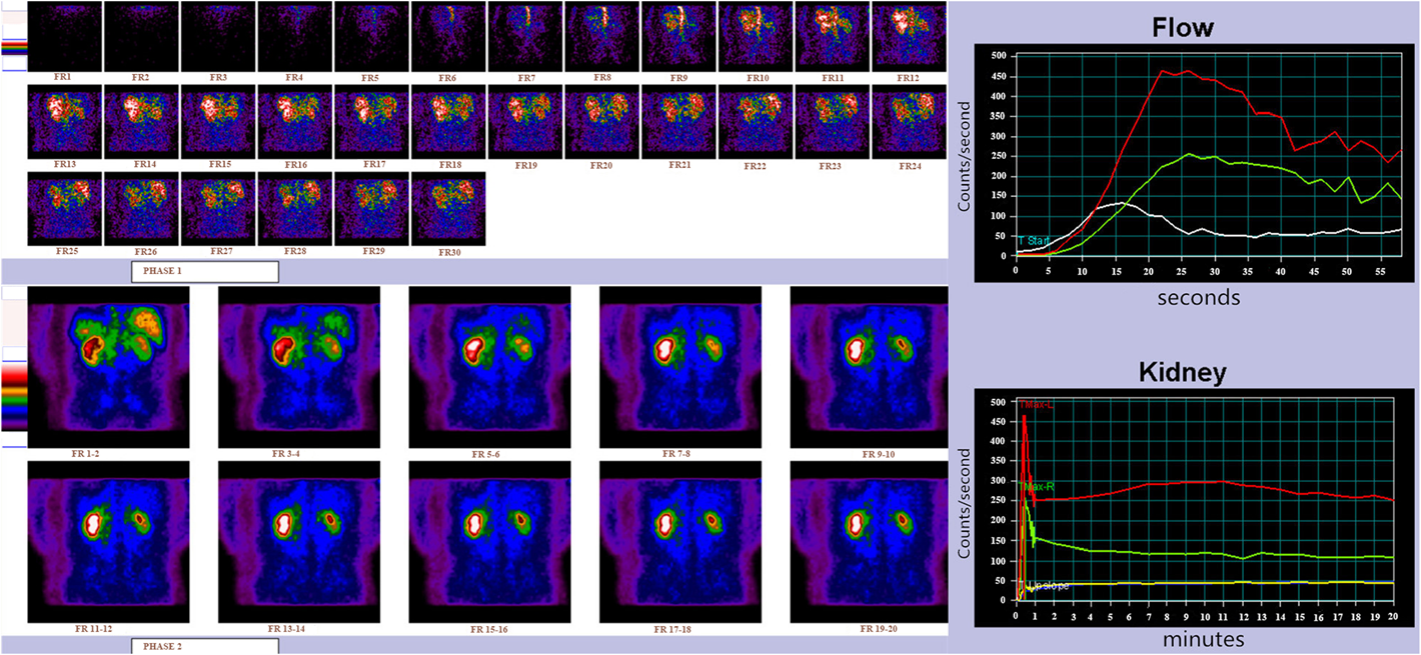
Dynamic renal scintigraphy for a critical patient by PICC. A 65-year-old man with absence of urine for 3 days; he was diagnosed with AKI stage 3, lung infection, atelectasis, electrolyte disorders, and coagulopathy, and the CRRT was initiated. On the fifth day, the patient was performed with endotracheal intubation and ventilator-assisted mechanical ventilation due to dyspnea and hypoxemia. A dynamic renal scintigraphy of 99mTc-DTPA was performed by PICC in this critical patient with AKI and artificially assisted ventilation. The relative renal uptake of the right kidney was 22,737 counts, the left kidney was 40,310 counts, the right kidney GFR was 14.6 mL/min, and the left kidney GFR was 25.9 mL/min. The bilateral renal blood flow significantly reduced and the renogram curves shown a renal failure pattern; the relative renal uptake of each kidney indicated the split renal function, respectively. These findings were most consistent with renal causes of acute renal failure
